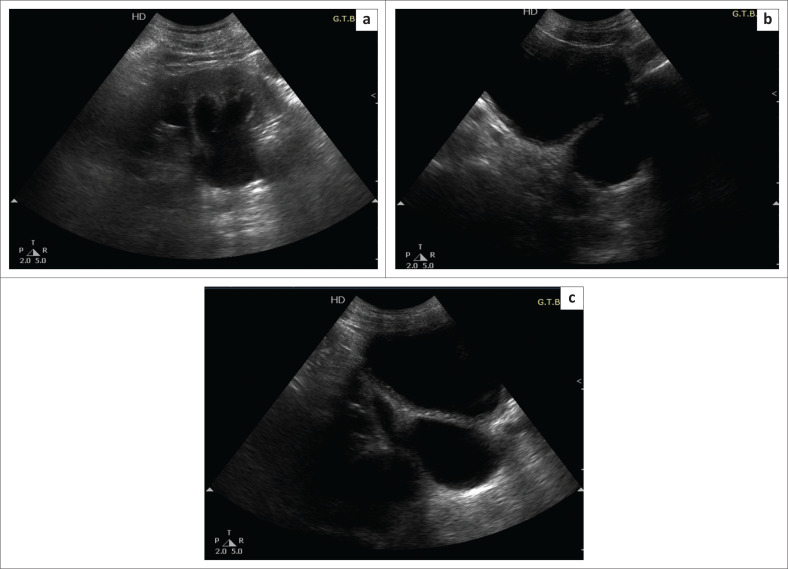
Ultrasound images show (a) gross hydronephrosis affecting the left kidney. (b) A well-defined outpouching in region of left vesicoureteric junction. (c) The left ureter is seen to open into the outpouching. Distal ureter appears dilated.
